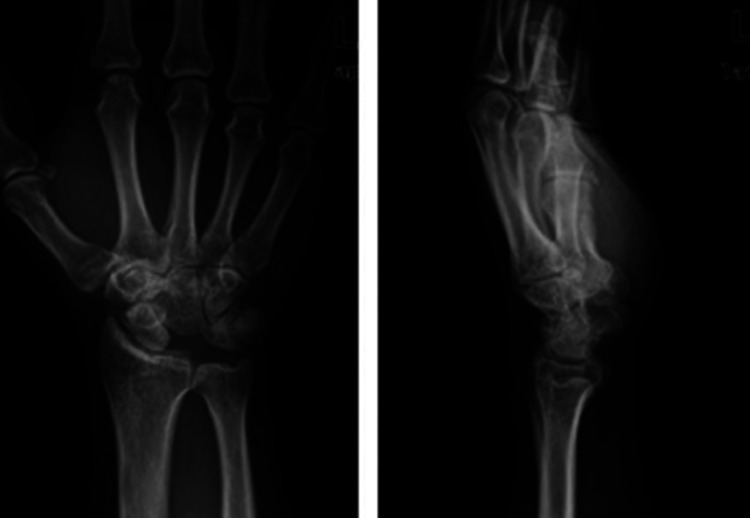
Postoperative X-ray after excision
